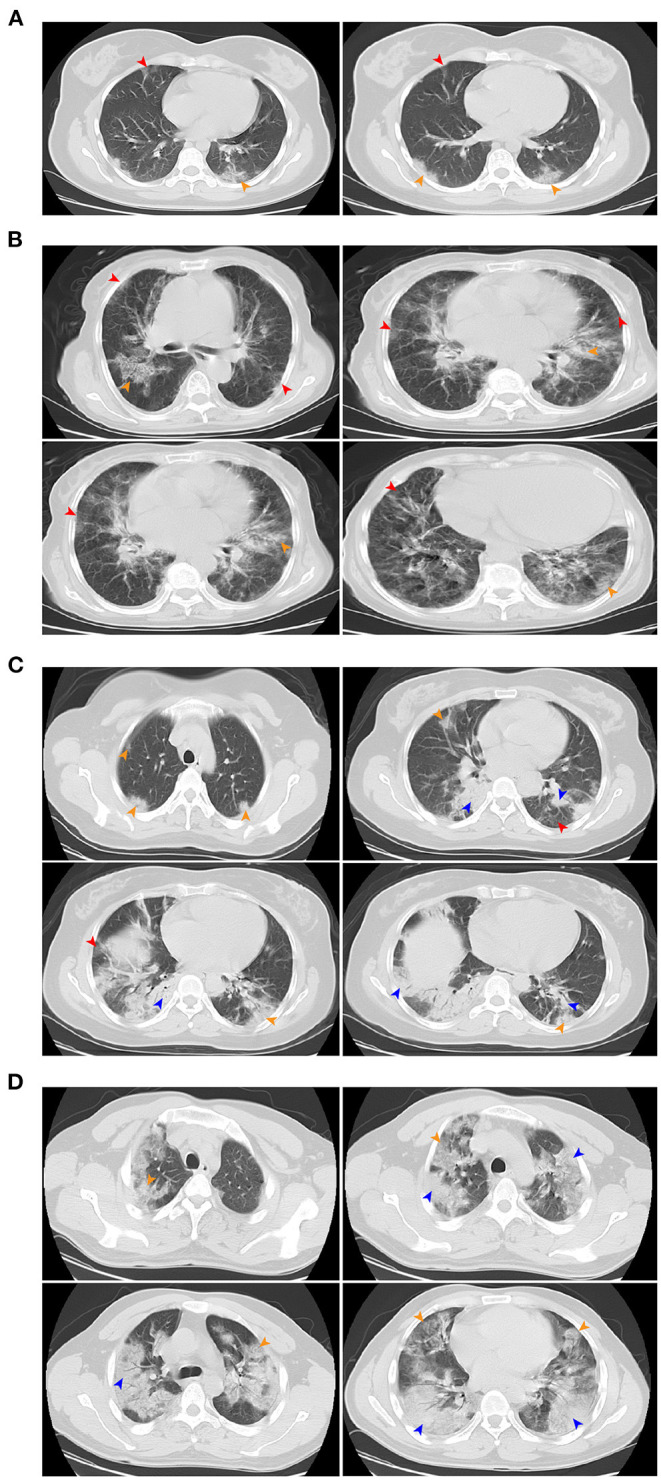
Chest CT images. (A) Chest CT images showing bilateral ground-glass opacity on day 5 after symptom onset. (B) Chest CT images showing bilateral ground-glass opacity on day 3 after symptom onset. (C) Chest CT images showing bilateral ground-glass opacity and bilateral subsegmental areas of consolidation on day 5 after symptom onset. (D) Chest CT images showing bilateral multiple lobular and subsegmental areas of consolidation on day 10 after symptom onset. (A,B) were mild pneumonia, (C,D) were severe pneumonia. Red arrow indicates ground-glass opacity, yellow arrow indicates nodular consolidation, blue arrow indicates the mixture areas of ground-glass opacity and nodular consolidation.
